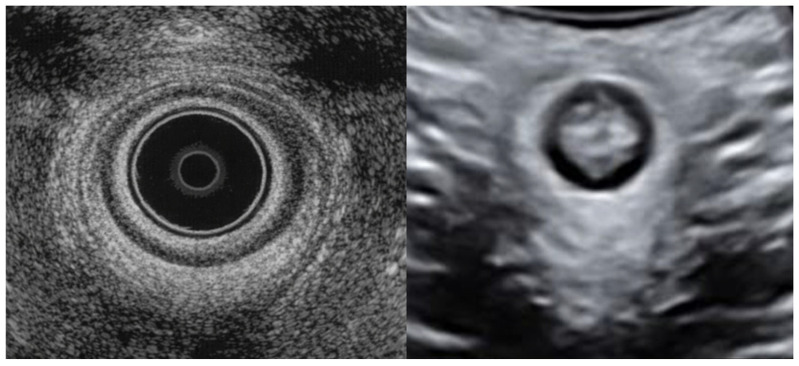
Representative endoanal (EAUS) and transperineal (TPUS) ultrasound images demonstrating normal anal sphincter anatomy.
